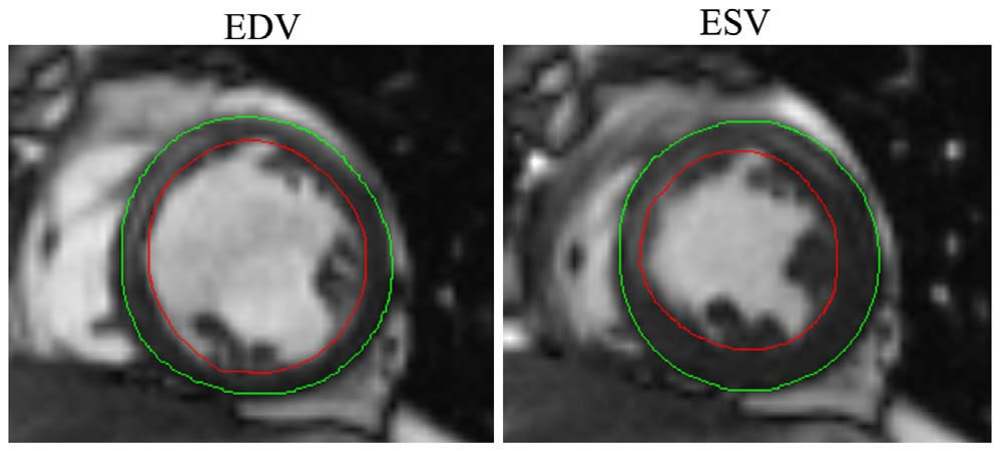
The delineations for the modalities are shown on short axis slices of MRI (end-diastolic and end-systolic).
